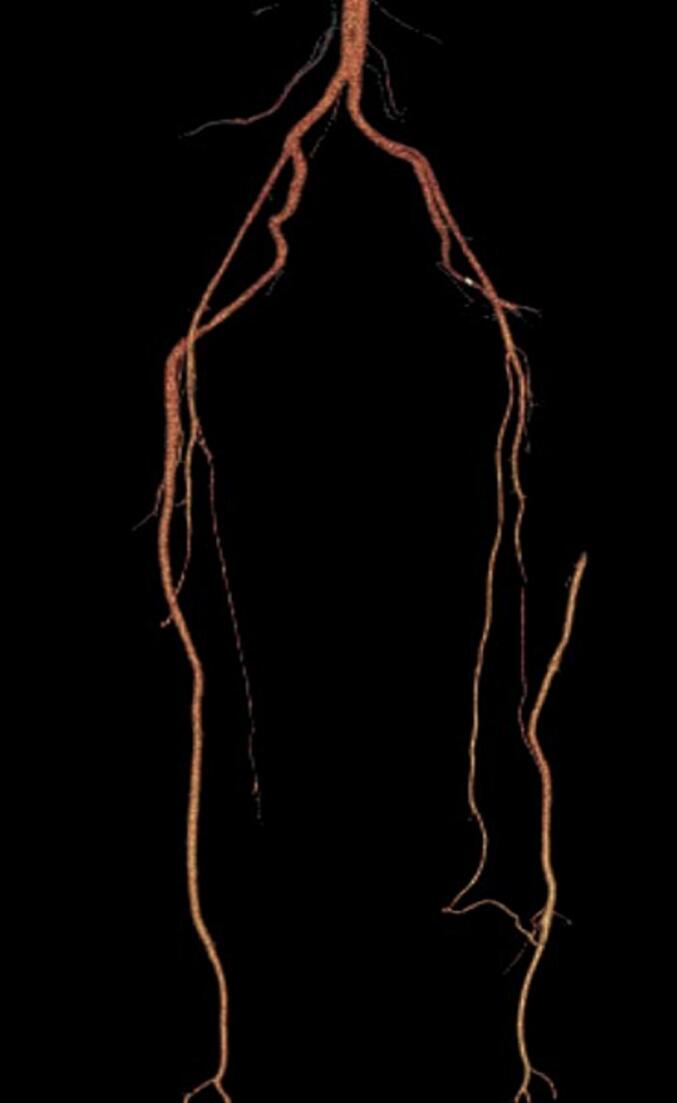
Coronal view 3D CT reconstruction of the arteries from hip to thigh, demonstrated hypertrophy of right sciatic artery and thrombosis proximal of left sciatic artery, mid part filled with collateral vessels.
